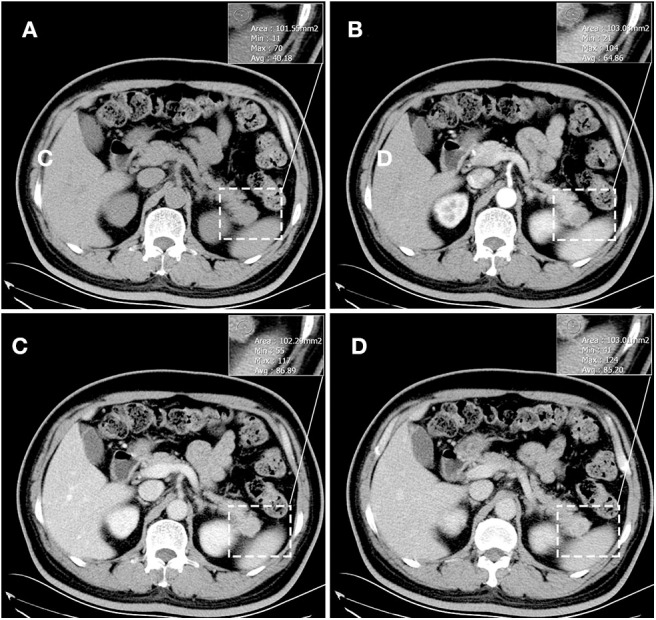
Unenhanced CT showed the mass to be isoattenuating and barely perceptible. (A) It demonstrated mild enhancement in the arterial phase (B) and moderate heterogeneous enhancement in the portal (C) and the late phases (D). The corresponding CT values were measured (insert). There was no evidence of superior mesenteric artery or portal venous invasion.
